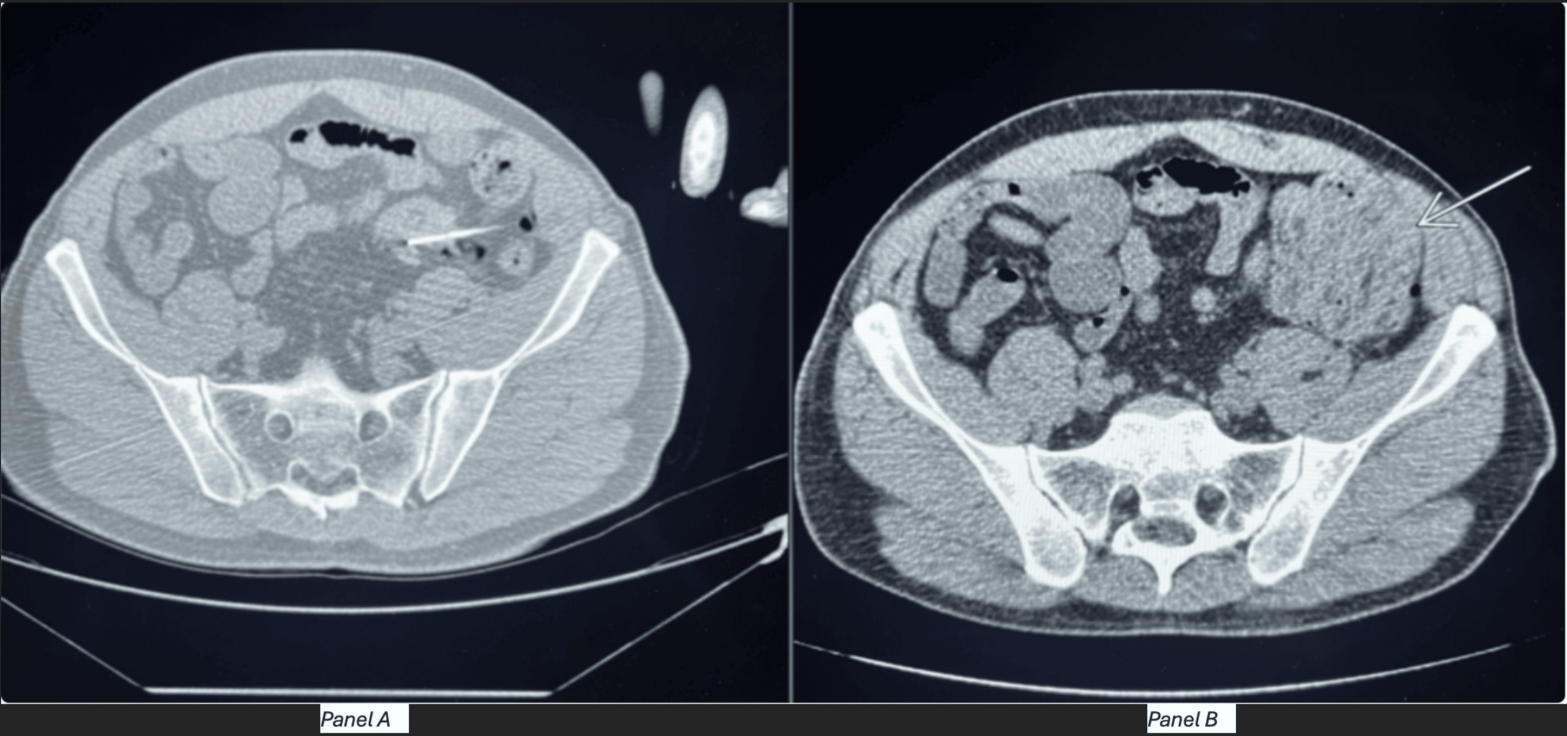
CT scan taken during (panel A) and after (panel B) the biopsy. Panel A: CT-guided needle biopsy. Panel B: Arrow pointing to subsequent hematoma formation following CT-guided needle biopsy.
